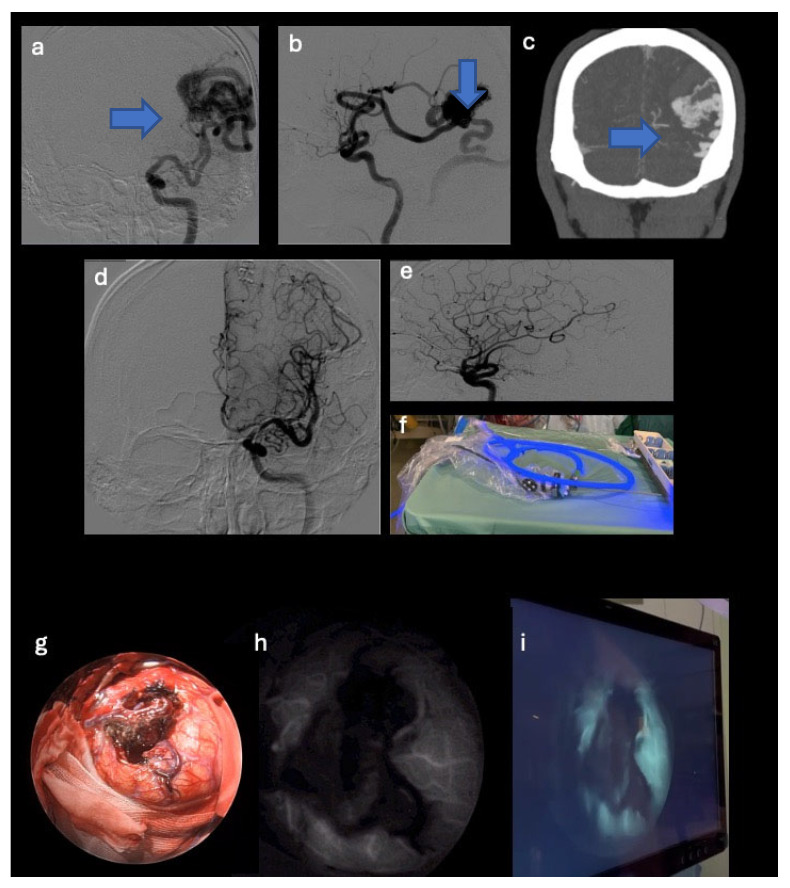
Preoperative digital subtraction angiography and computed tomography angiography images that show an arteriovenous malformation (blue arrows) originating from the left middle cerebral artery and venous drainage into the ipsilateral transverse sinus (a–c). Postoperative digital subtraction angiography images that highlight the exclusion of the nidus (d,e). Endoscope equipment (f), standard endoscopic view (g), and endoscope in the SPY mode ready for intraoperative use demonstrating the arteriovenous malformation exclusion (h,i).
